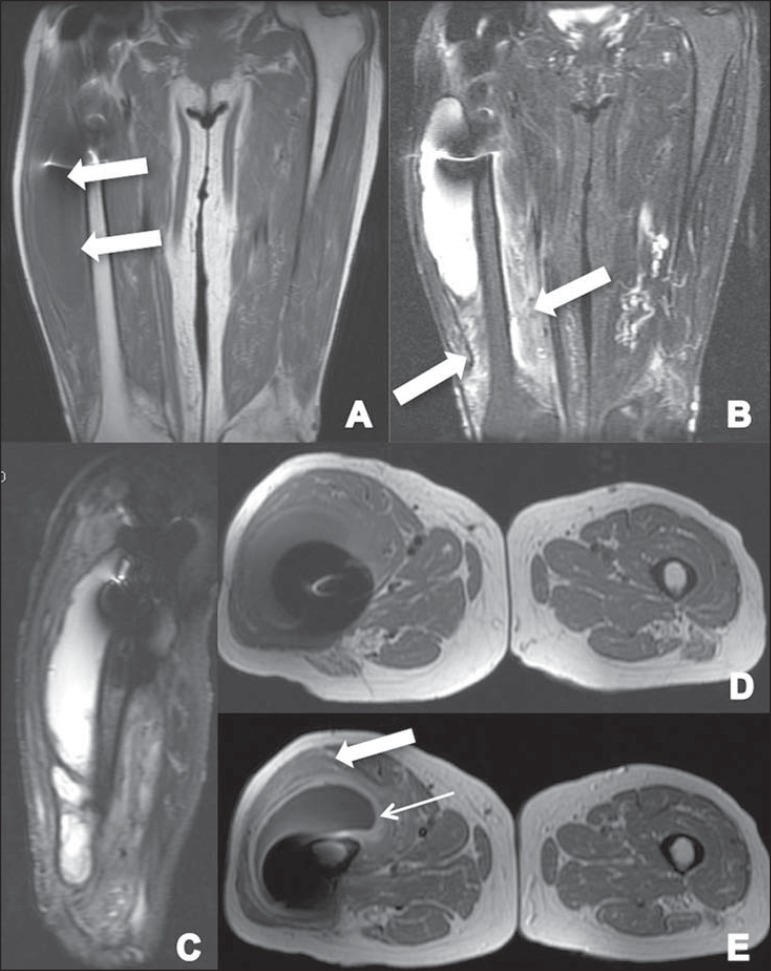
A: Coronal T1-weighted section showing the presence of a
